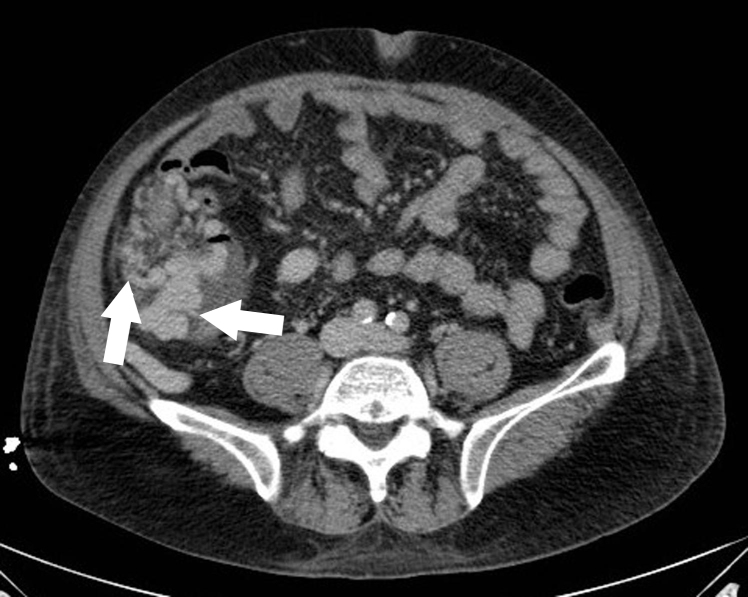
Computed tomography, axial view. Arrows indicate varices.
